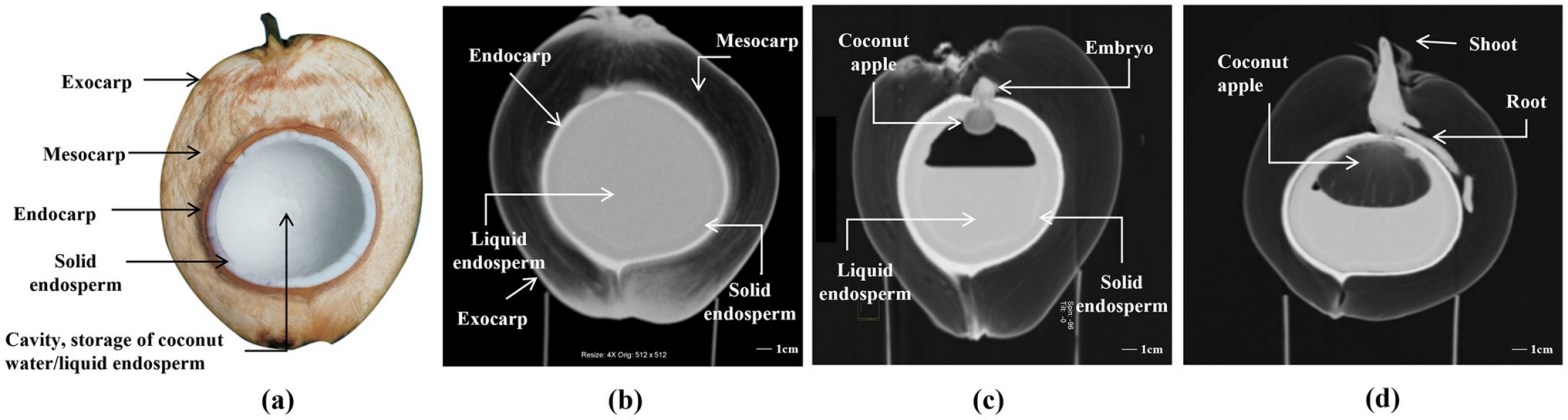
A physical photo of cut coconut and three computed tomography multiplanar reformation images of different coconuts.
